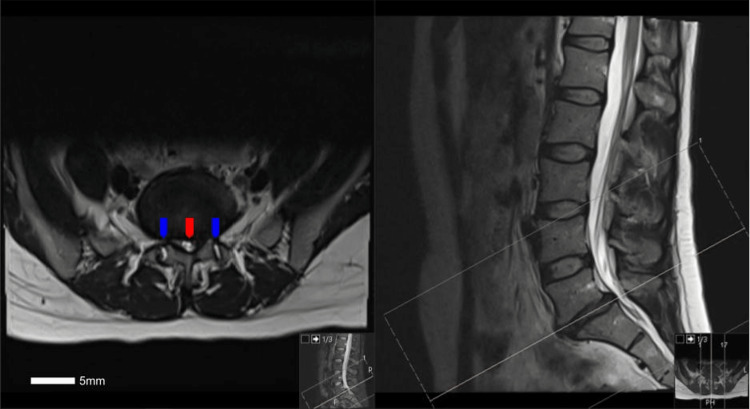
Axial (left) and sagittal (right) T2-weighted MRI of the lumbar spine without contrast at the level of L5-S1, demonstrating severe central canal and lateral recess stenosis (red arrow) with bilateral foraminal stenosis, as well as facet joint effusions (blue arrows)
